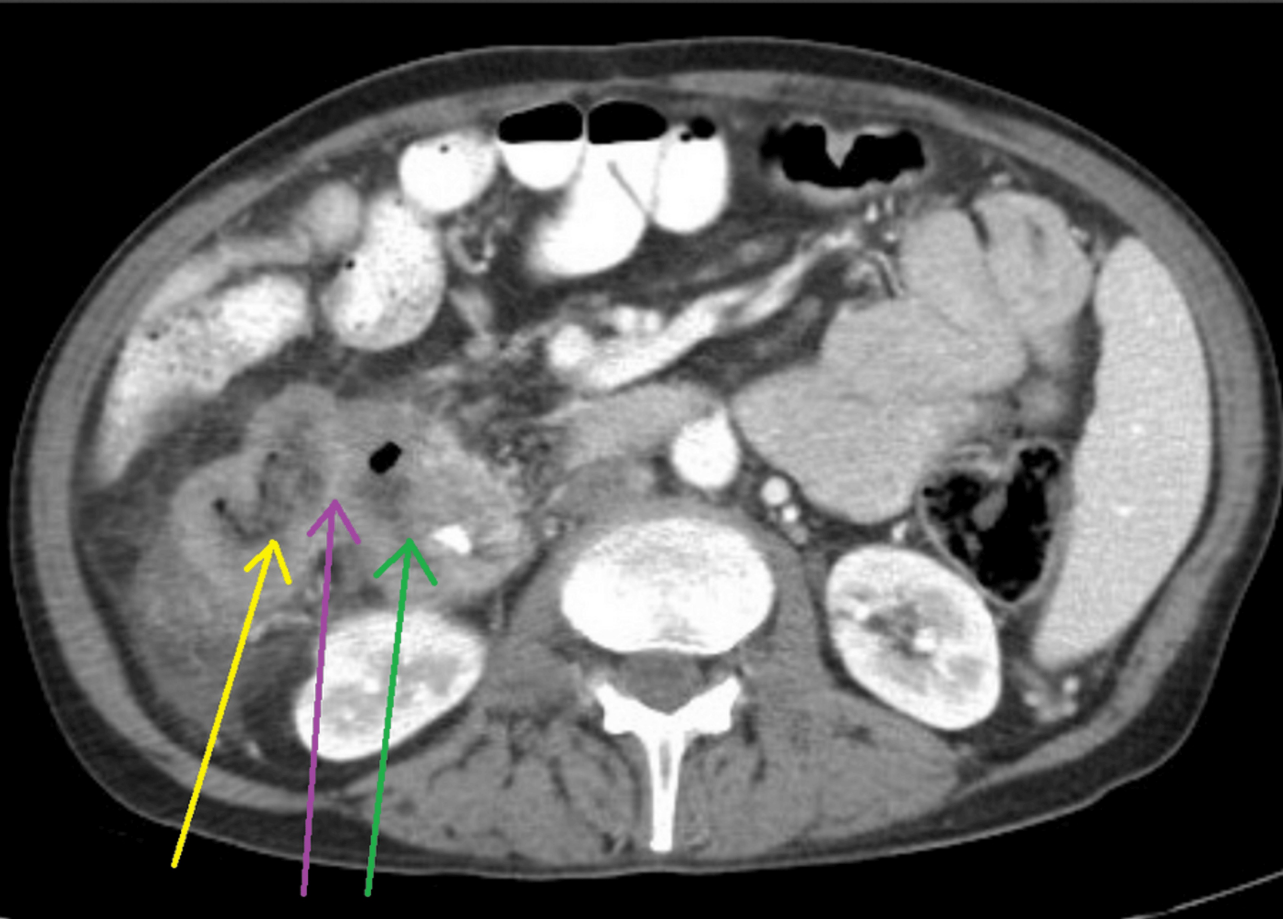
Abdominal CT image demonstrating a fistulous communication (purple arrow) between the duodenum (green arrow) and the adjacent ascending colon (yellow arrow)
